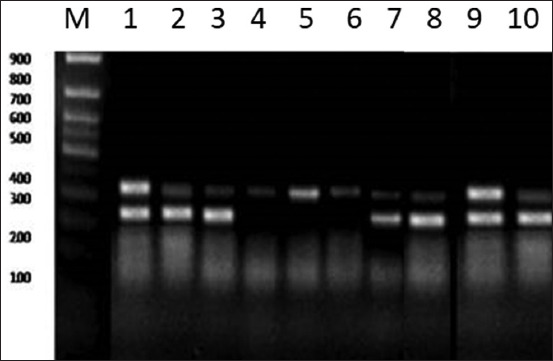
DNA electrophoretic pattern of diacylglycerol acyltransferase 1 amplicon after digestion with *Alu1* endonuclease. Lane M: 100 bp DNA ladder, Lanes genotype CC (4, 5, 6) (309 bp) and Lanes (1, 2, 3, 7, 8, 9, 10) genotype CT (309, 272, and 37 bp).
